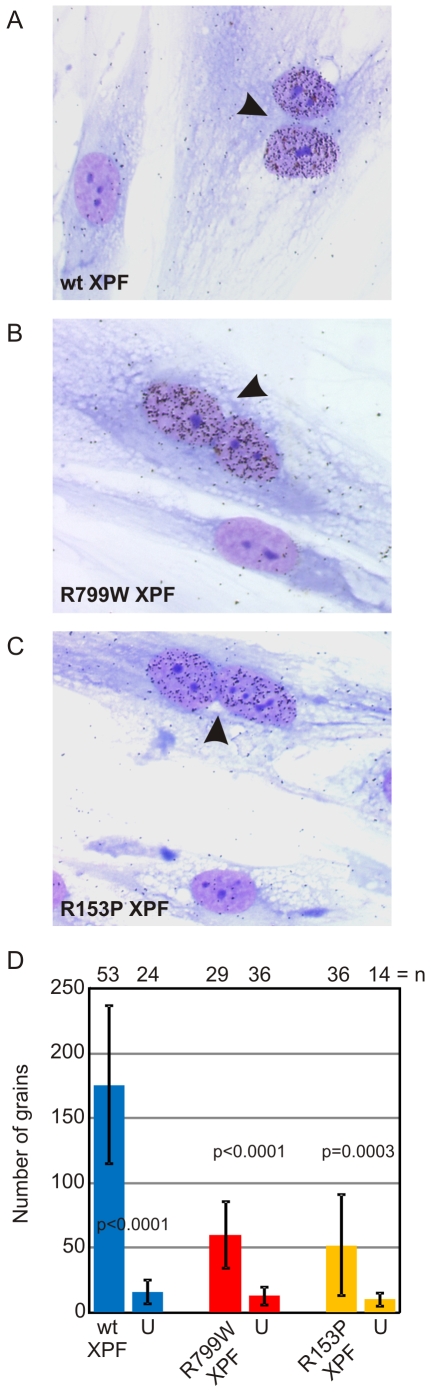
Correction of *XPF* mutant cell NER defect by microinjection of XPF-ERCC1. Primary fibroblasts from XFE progeroid patient XP51RO were fused to create homopolykaryons by treatment with inactivated Sendai virus then plated on glass coverslips. Only homopolykaryons were injected with recombinant XPF-ERCC1 protein complex (A) wild-type (B) XPF^R799W^-ERCC1 (C) XPF^R153P^-ERCC1. The cultures were irradiated with 10 J/m^2^ UV-C and ^3^H-thymidine was added to the culture. UV-induced unscheduled DNA synthesis was detected by autoradiography. Homopolykaryons are indicated with arrows. (D) Histogram indicating the average number of radiographic grains in nuclei injected with each of the recombinant protein complexes and uninjected cells in the same sample. Error bars indicate the standard deviation. N indicates the number of nuclei analyzed in each population. P values for the comparison between injected and uninjected cells were calculated using an unpaired two-tailed Student's t-test.
